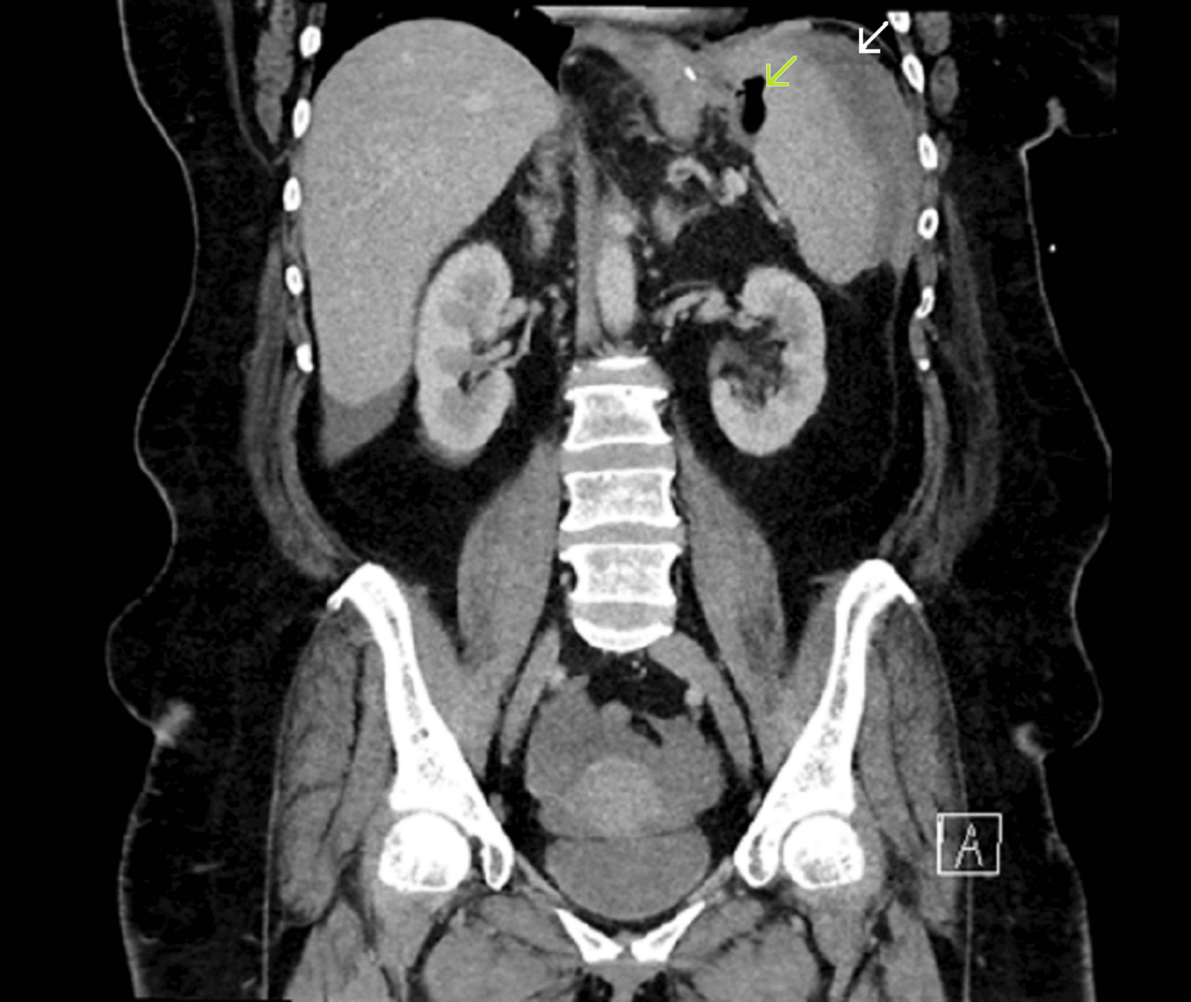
Index CT scan on presentation to ED Green arrow indicating gas surrounding splenic hilum. White arrow indicating intra-abdominal free fluid. ED, emergency department; CT, computed tomography
